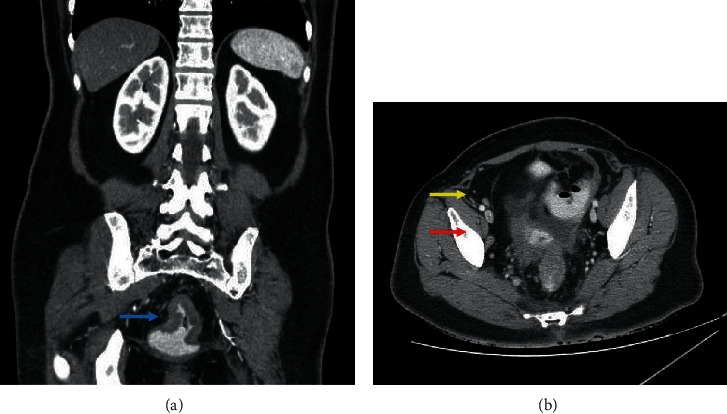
CT of the abdomen and pelvis in coronal view (a) showing a rectal mass and in axial view (b) showing small noncalcific right internal (red arrow) and right external (yellow arrow) iliac lymph nodes.
